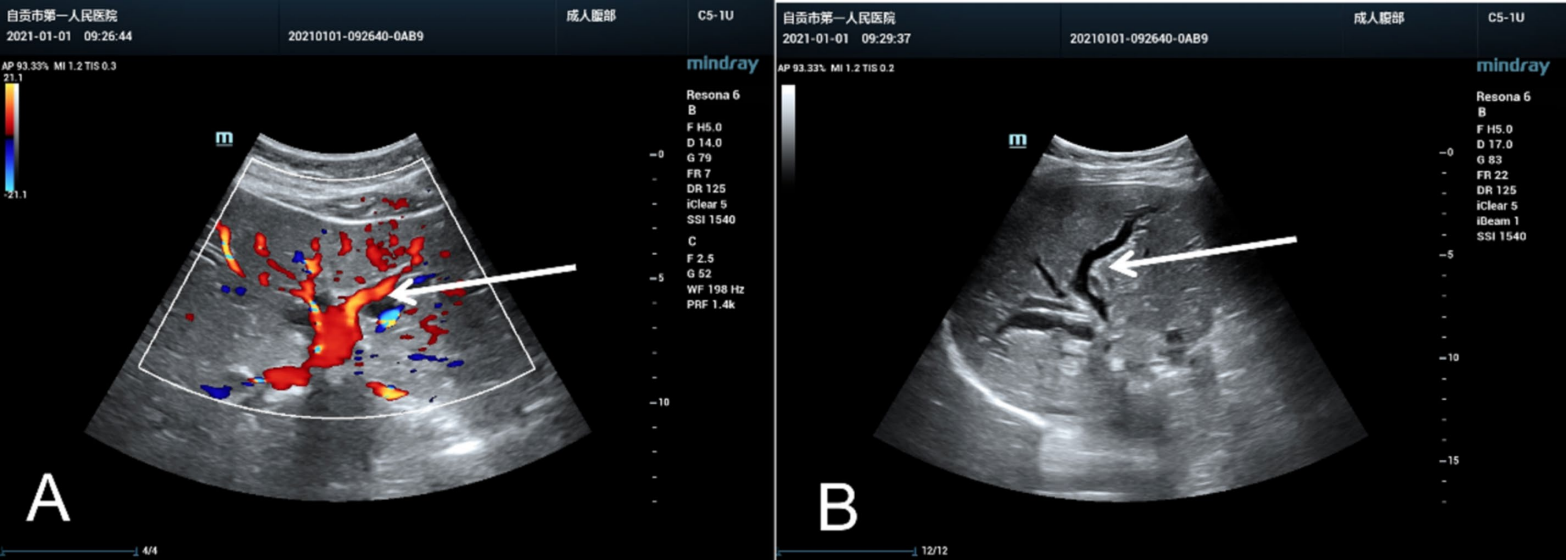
The ultrasound images of the liver resembled a spider or an evil spirit walking in the hepatic hilum of the pregnant woman. The oblique diameter of the right liver was approximately 14.5 cm. There were echoless areas in the liver, and the larger one was approximately 2.2×1.5 cm. The liver capsule was not smooth, the internal diameter of the main portal vein was approximately 1.0 cm, and dilatation of the bile duct was evident without mass effect in the hepatic hilar. (A) Color Doppler ultrasound image and (B) Two-dimensional ultrasound image, marked with a white arrow.
